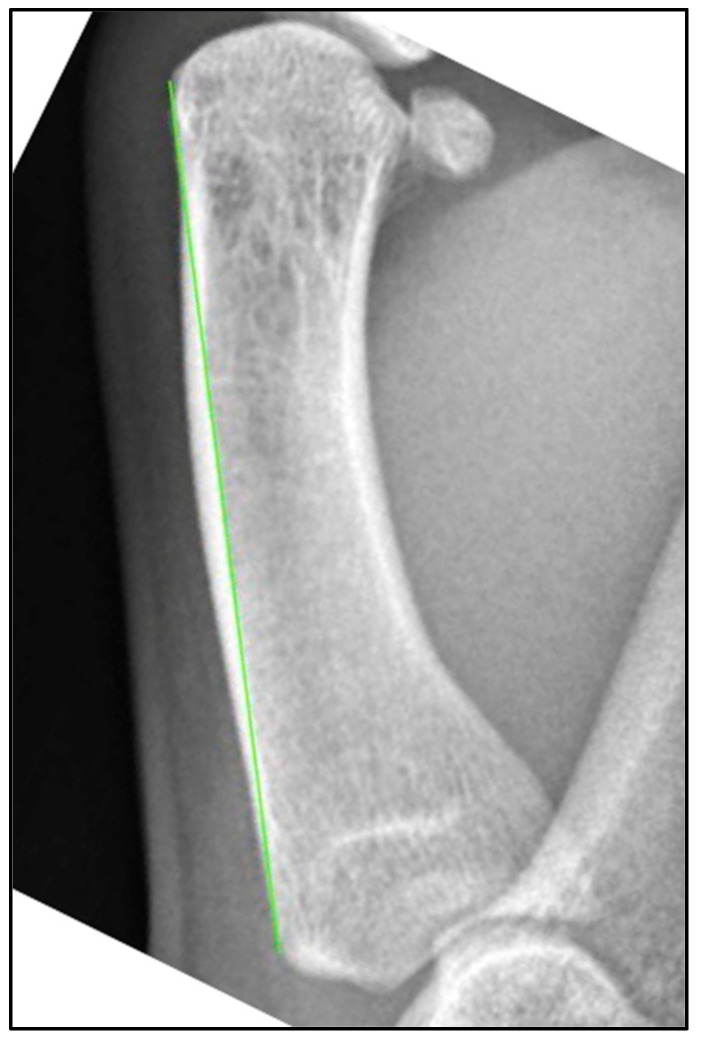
Lateral radiograph of a first metacarpal bone showing uneven dorsal cortex shape.
